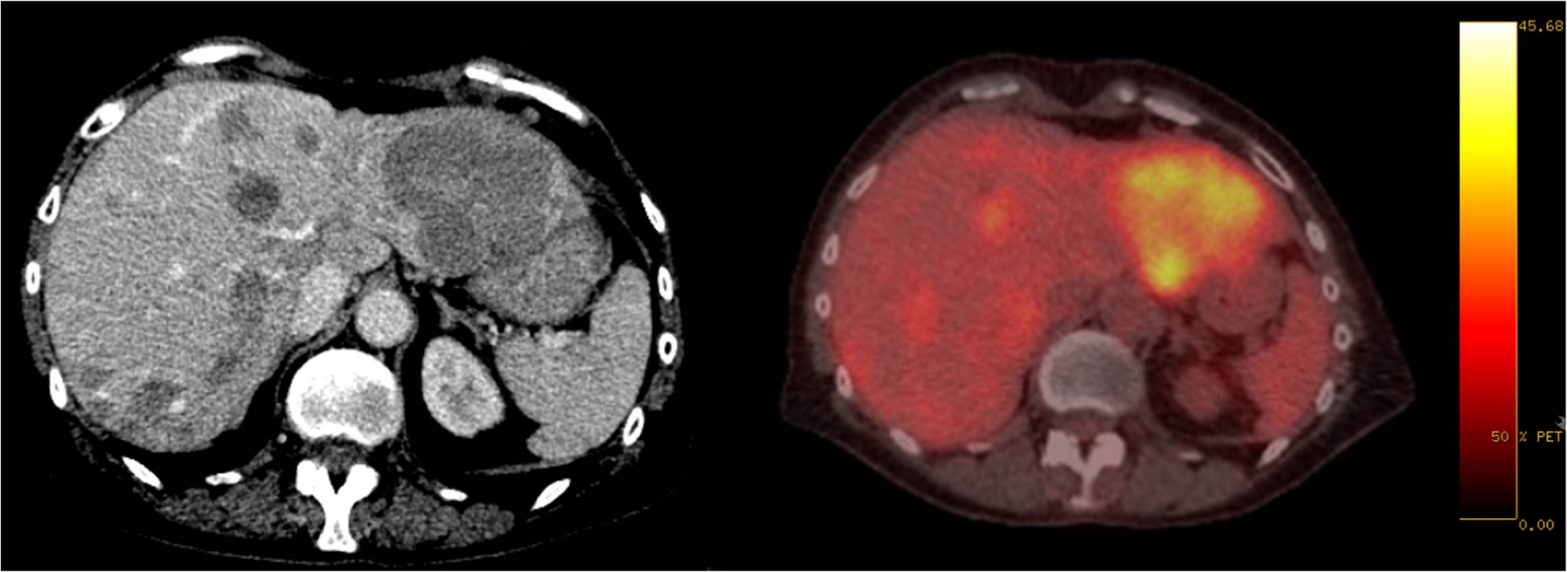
Computed tomography scan (*left*) and ^18^fluorodeoxyglucose-positron emission tomography scan (*right*) (background blood pool maximum standardized uptake value 1.5, hepatic maximum standardized uptake value 2.6) showing updated cross-sectional imaging of the patient’s liver heavily infiltrated with metastases from neuroendocrine tumor
